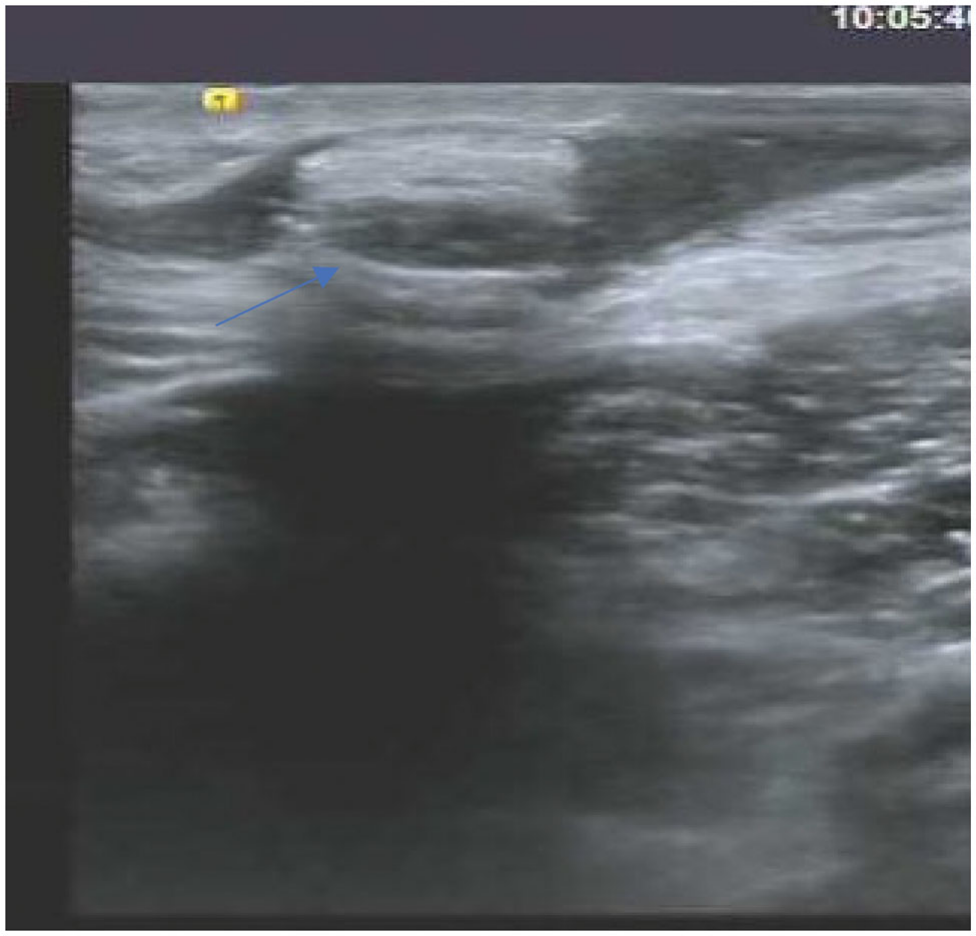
B-Mode Ultrasound Image of case 3.
